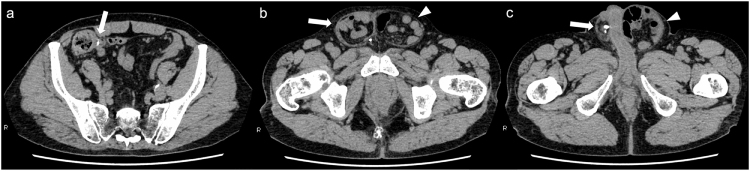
Axial computed tomography scan of the abdomen before the second surgery. (a) Appendiceal root dissected during the first surgery (arrow). (b) Right inguinal hernia (arrow) and left inguinal hernia (arrowhead). (c) Right inguinal hernia containing the appendiceal remnant from the first surgery (arrow) and left inguinal hernia (arrowhead).
